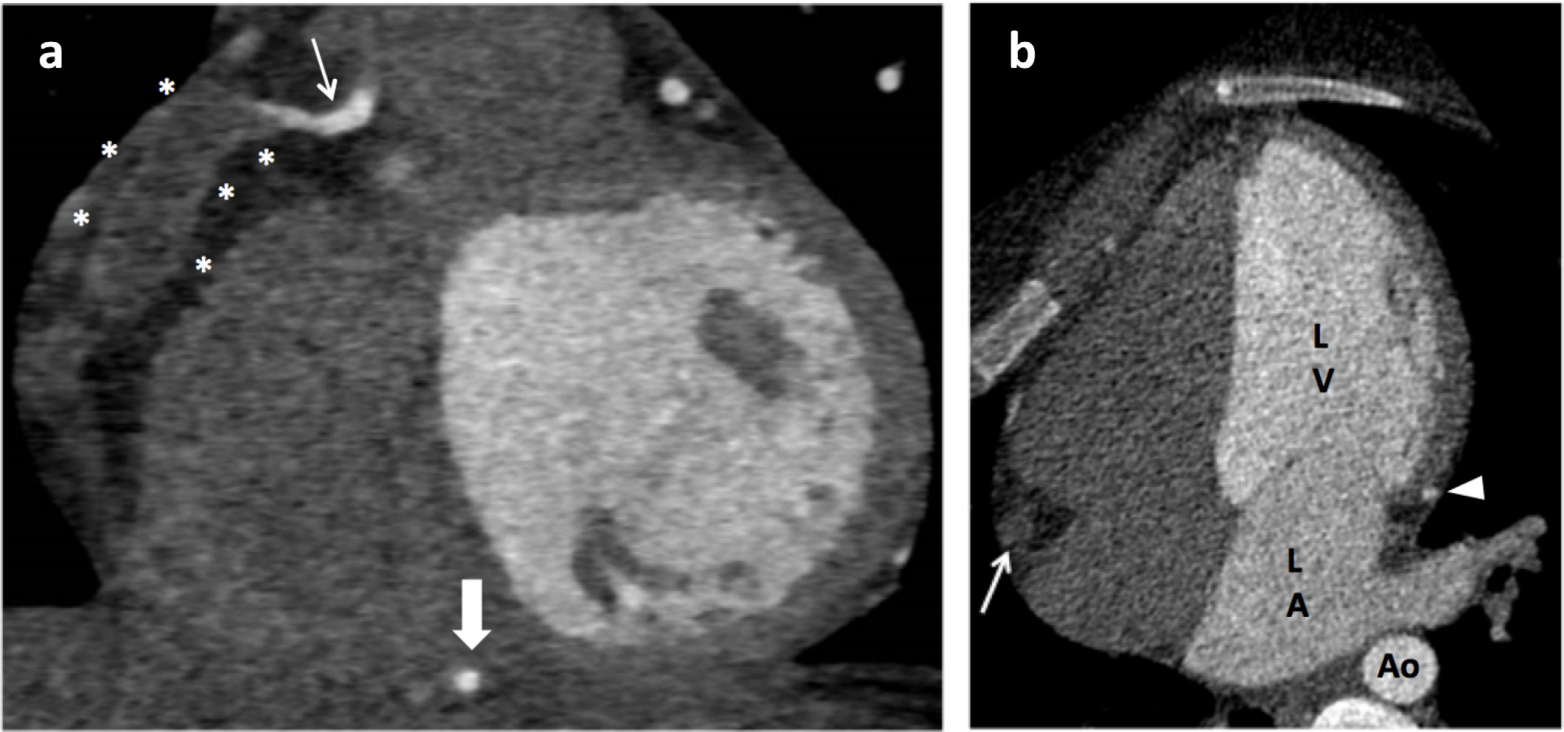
CT coronary angiogram demonstrating a right coronary artery dissection.* 1 .a—coronal slice demonstrating the right coronary artery within the atrioventricular groove. The proximal right coronary artery is normal calibre and opacified with contrast suggesting patency (thin arrow). The patent vessel then tapers into a non-opacified dissected vessel expanded with thrombus (stars). There is distal refill of the PDA branch (thick arrow) via collaterals. 1.b—four chamber view demonstrating the right coronary artery expanded with thrombus (arrow). The left circumflex coronary artery is normal calibre and opacified with contrast suggesting patency (arrow head). Ao, aorta; LA, left atrium; LV, left ventricle.
